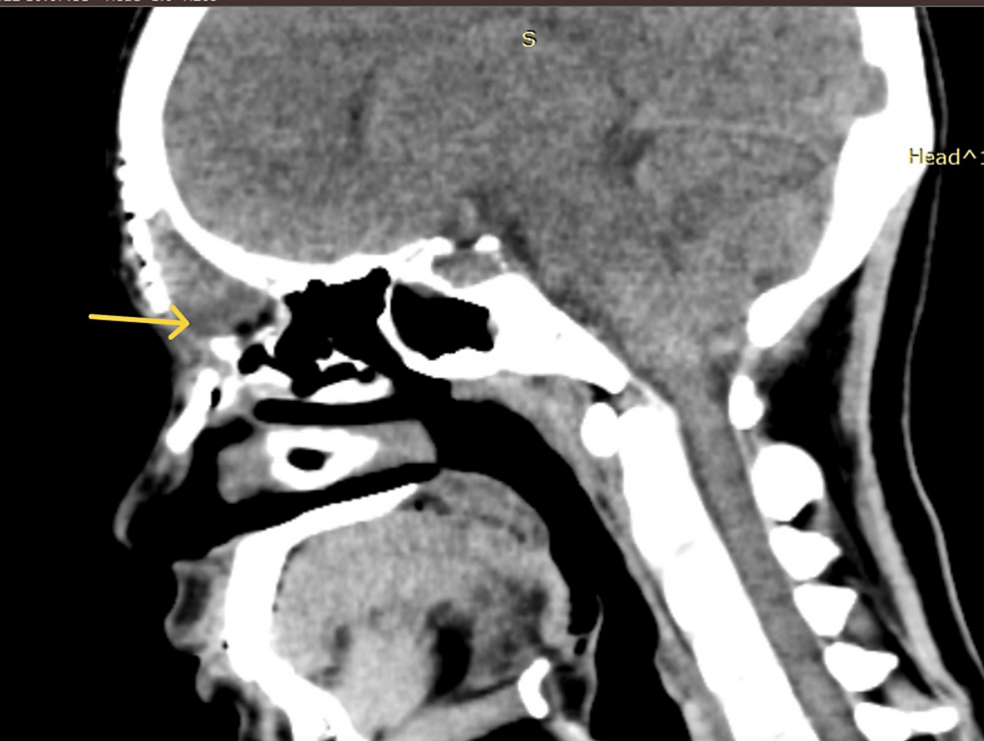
Sagittal view of CT PNS done on the 11th postoperative day showing a defect in the anterior bony wall of the right frontal sinus with collection in the sinus cavity. CT, Computed tomography; PNS, Paranasal sinus.
